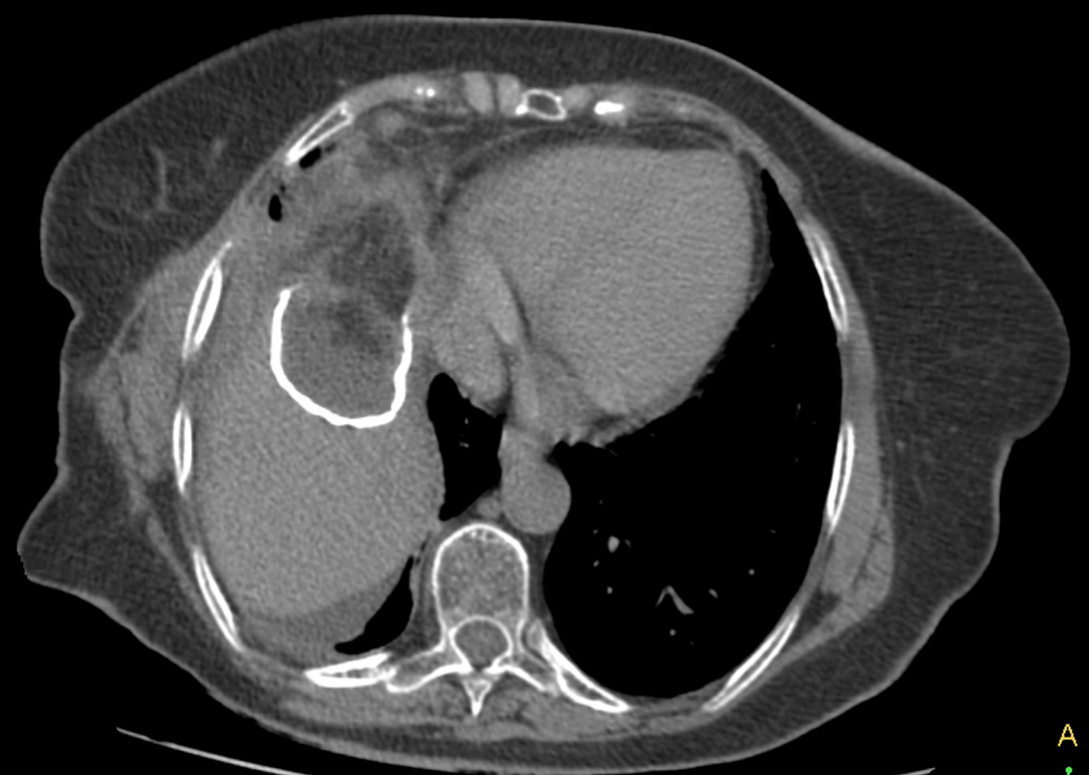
CT scan 2 months postoperatively showing the remnant calcifications of the cyst, the omental patch roofing it, and growing of hepatic tissue around the calcified wall (white arrow).
